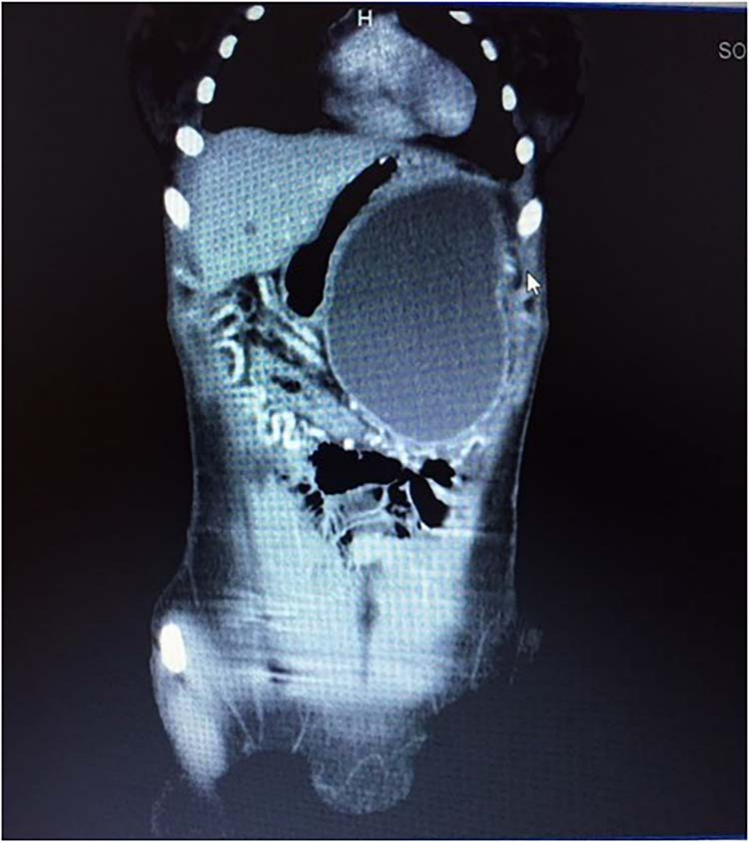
CT scan showing mass with well-defined borders.
